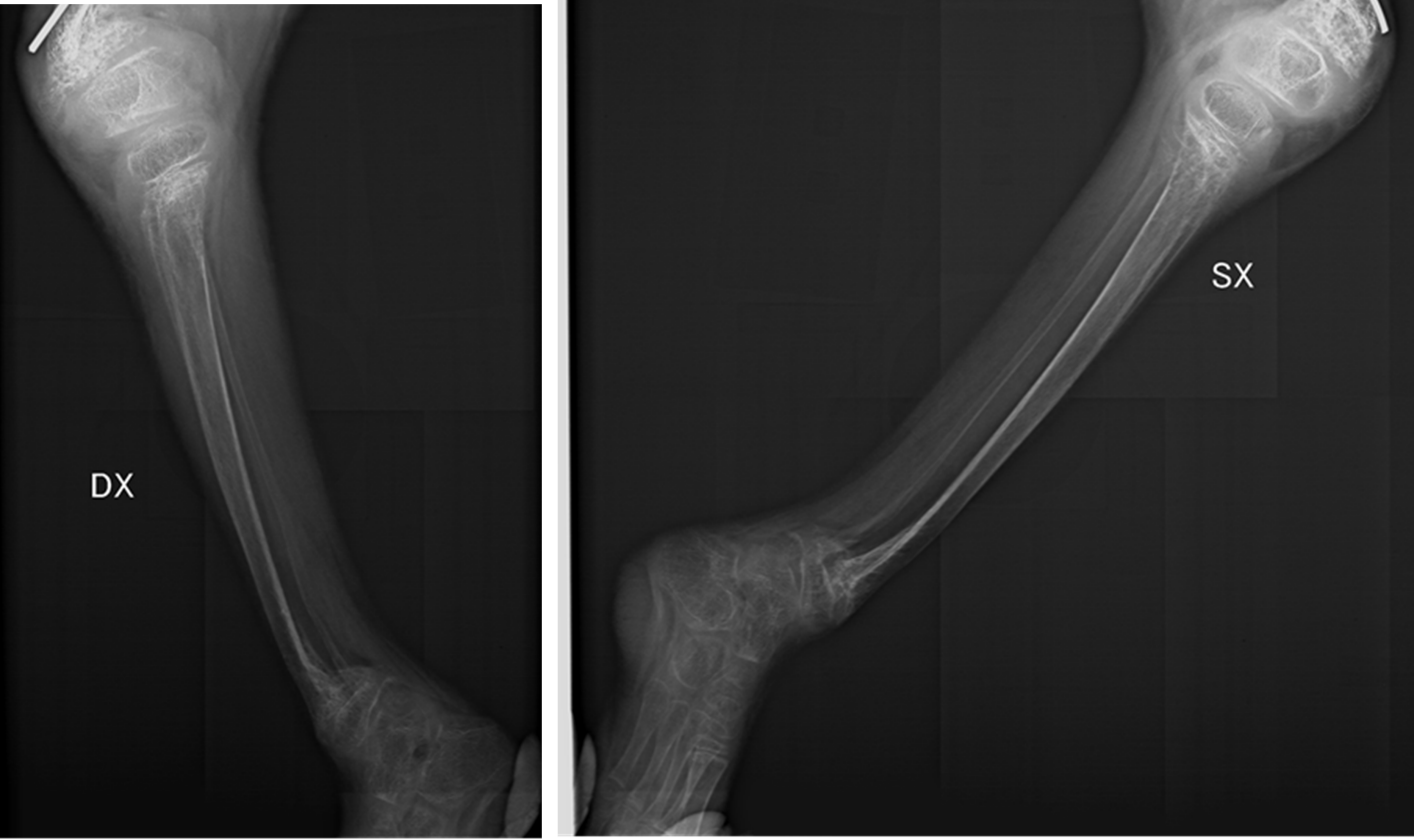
Tibia (radiographic image of right and left tibia dimorphism with angulated distal segments).
